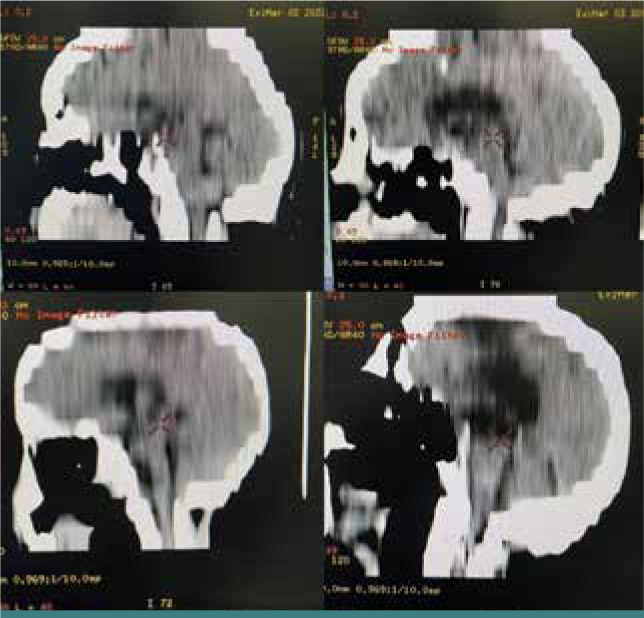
CT scan images with different pixel values
